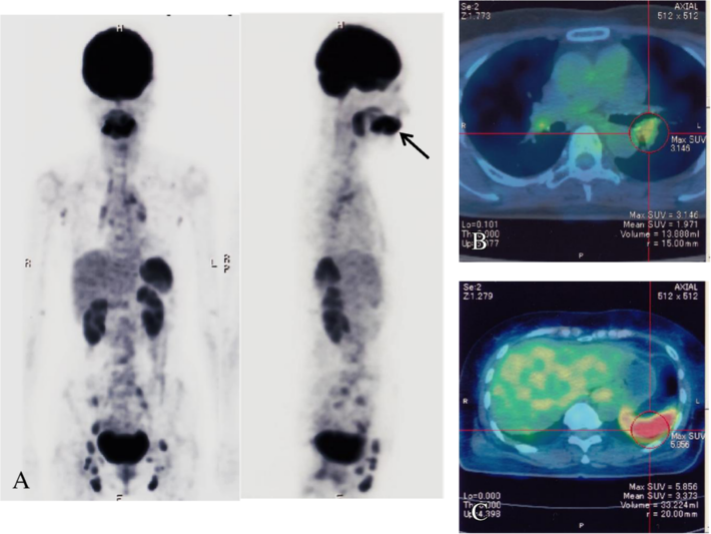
Positron emission tomography-computed tomography images on admission. (A) Coronal and sagittal positron emission tomography images on admission showing ^18^F-fluorodeoxyglucose uptake in peripharyngeal, axillary, mediastinal, hilar, iliac and inguinal lymph nodes and spleen. An arrow indicates ^18^F-fluorodeoxyglucose uptake at submandibular dental roots, suggesting submandibular periodontitis. (B) Fused positron emission tomography-computed tomography image showing left hilar lymph node swelling with moderate ^18^F-fluorodeoxyglucose uptake. (C) Fused positron emission tomography-computed tomography image showing remarkable ^18^F-fluorodeoxyglucose uptake in the spleen.
